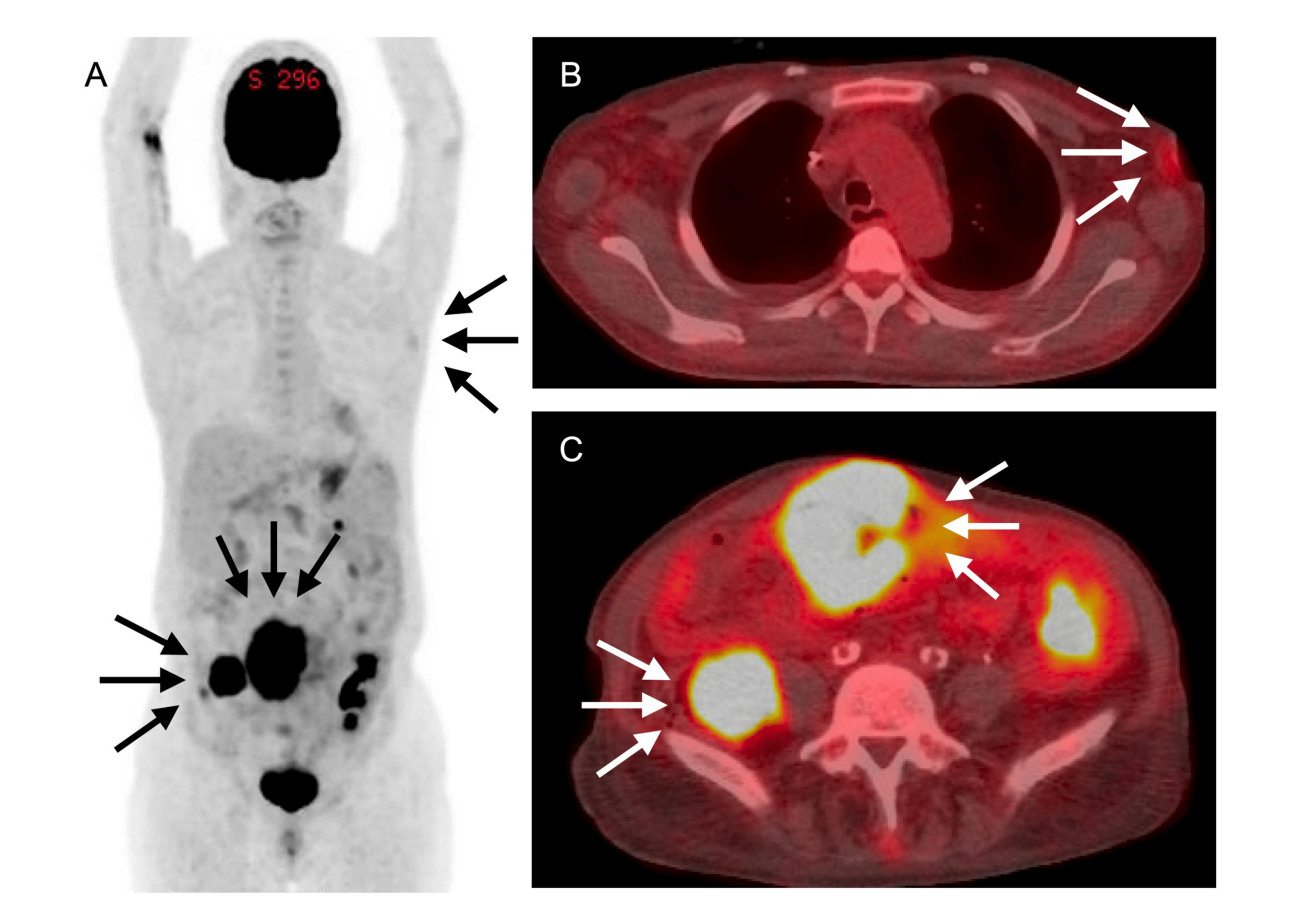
PET-CT findings. (A) Coronal section and (B) axial section of the thorax show FDG uptake in a left axillary mass. (C) The axial abdominal section shows intense FDG uptake in the thickened small intestinal wall immediately beneath the abdominal wall (SUVmax 17.4) and in the right retroperitoneal mass (SUVmax 10.0).
